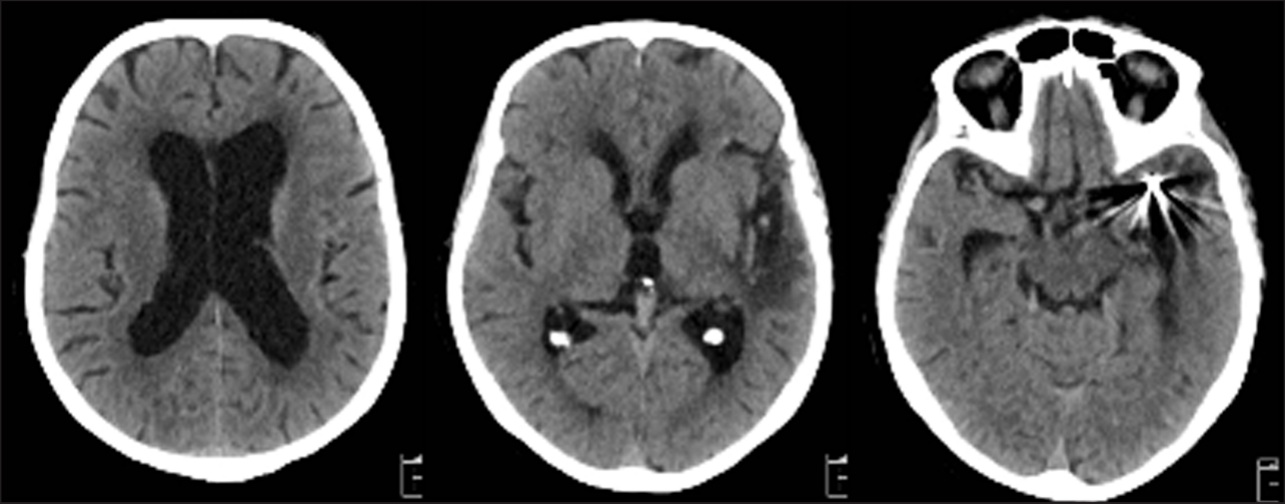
Unenhanced CT of the brain showed no abnormalities except known gliotic changes in the left temporo-insular region from an old haemorrhage and streaking artefacts from coiling material at the level of the left MCA-bifurcation.
